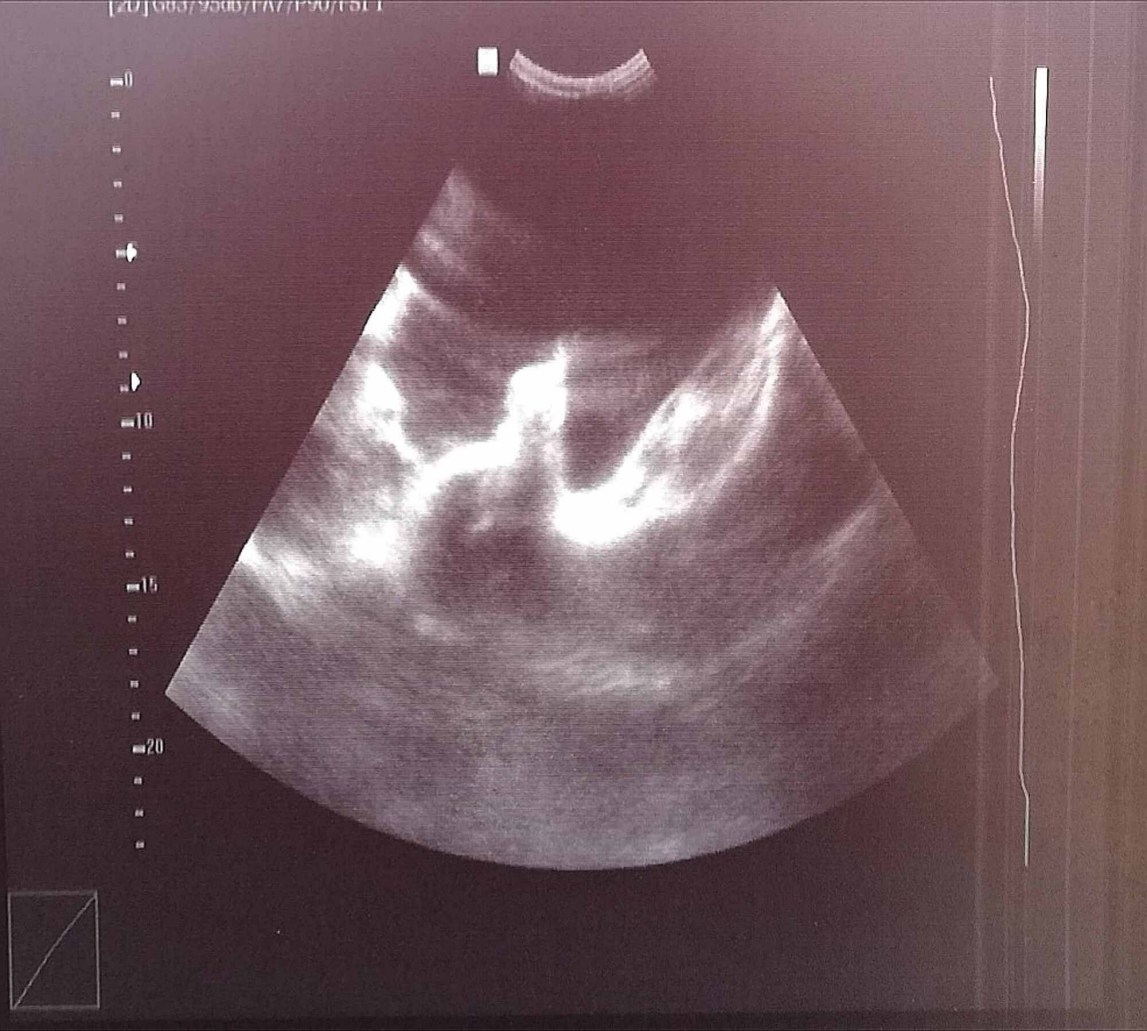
Ultrasound image showing free fluid in the pelvic cavity
